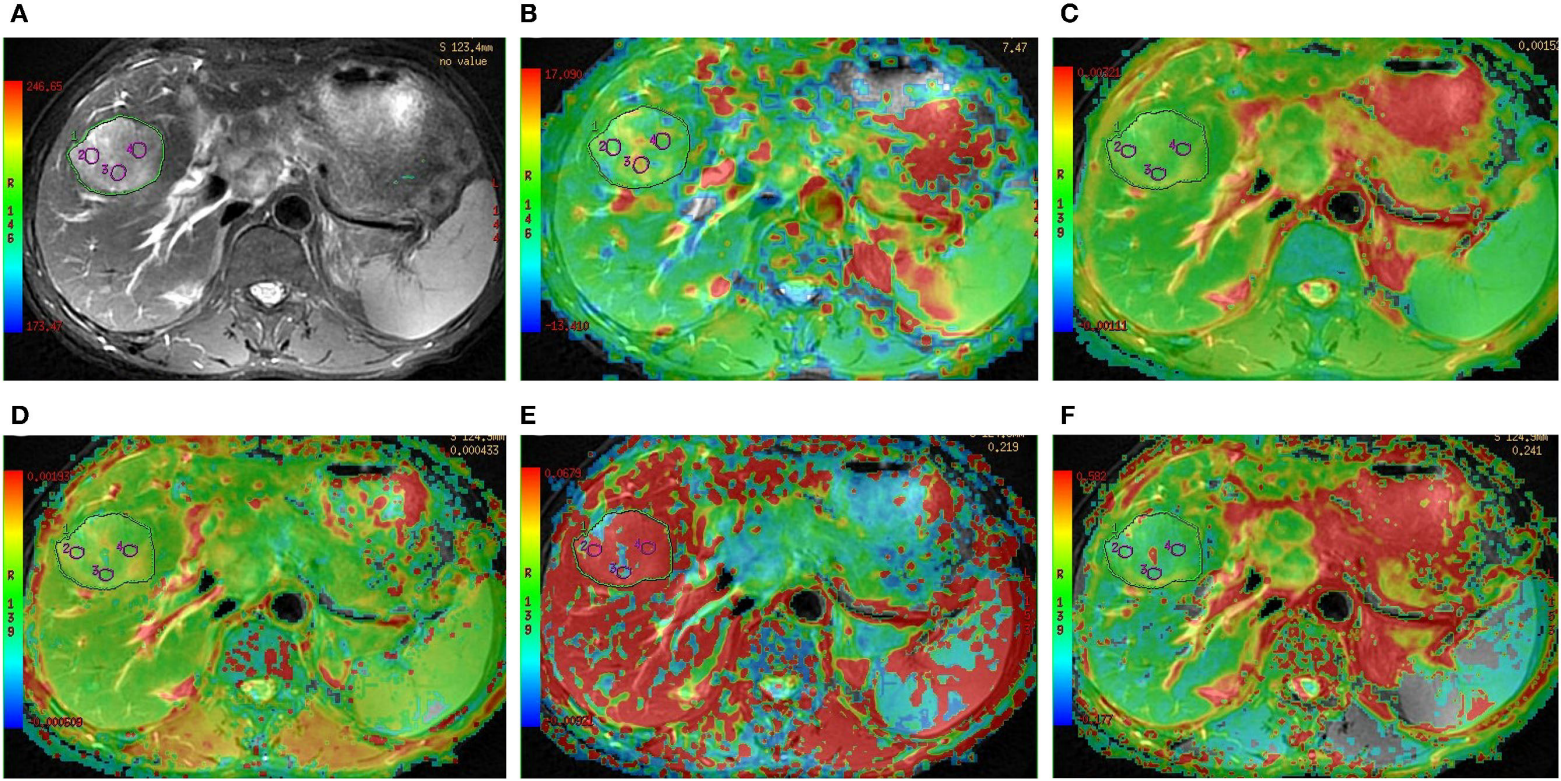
Preoperative MR images of one lesion in a 47-year-old man with grade 4 HCC. (A) T_2_-weighted image, (B) APT image of HCC fused with T_2_-weighted imaging indicates that the average APT SI value determined by two observers is 6.35%, (C–F) IVIM-derived pseudo-colored maps of HCC (ADC, D, D*, and *f* , respectively) fused with T_2_-weighted imaging indicate that the average ADC, D, D*, and *f* values determined by two observers were 1.14 × 10^−3^ mm^2^/s, 0.45 × 10^−3^ mm^2^/s, 98.41 × 10^−3^ mm^2^/s, and 27.52%, respectively.
